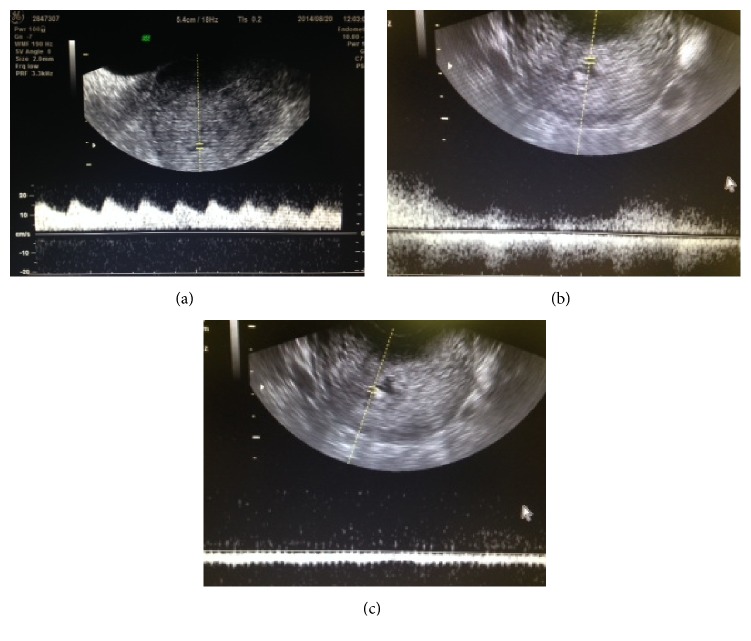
Diagnosis of AVM by Doppler examination (Patient 1). (a) Several arteries were observed in AVM. (b) A turbulent aspect in the fistula. (c) Venous flow in the intramural venous plexus.
